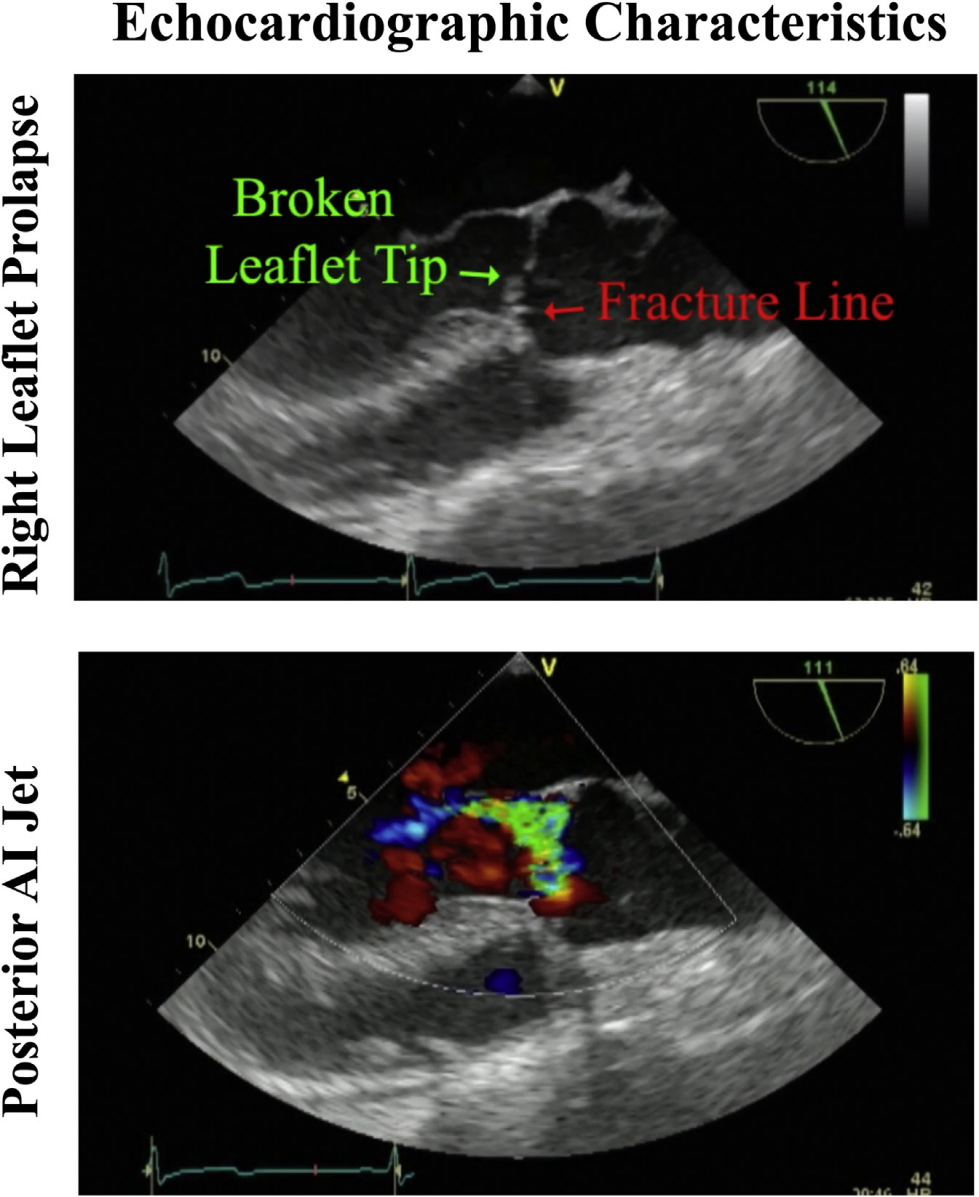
Typical echocardiogram of a patient with isolated right coronary leaflet prolapse showing a “broken” leaflet tip, a leaflet “fracture line,” and a posterior aortic insufficiency (*AI*) jet.
